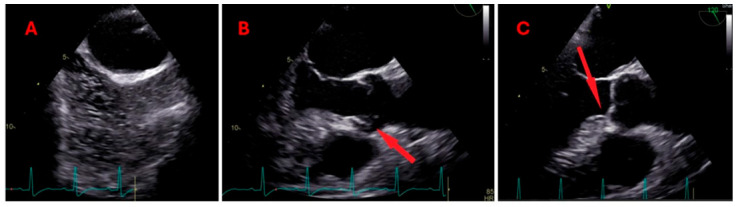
(A): Transthoracic echocardiography—bicav section: no detectable shunt at IV injection of bubbled glucose serum. (B,C): Transesophageal cardiac ultrasound (TEE)—Lambl excrescences at the level of the aortic cusps ((B)—parasternal long-axis view, (C) short axis level of the aortic valve).
